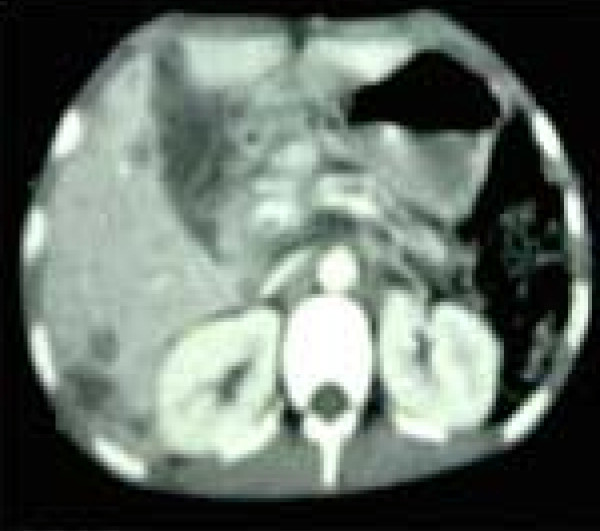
Computerized tomography (CT) scan revealing metastatic lesions of the liver.
